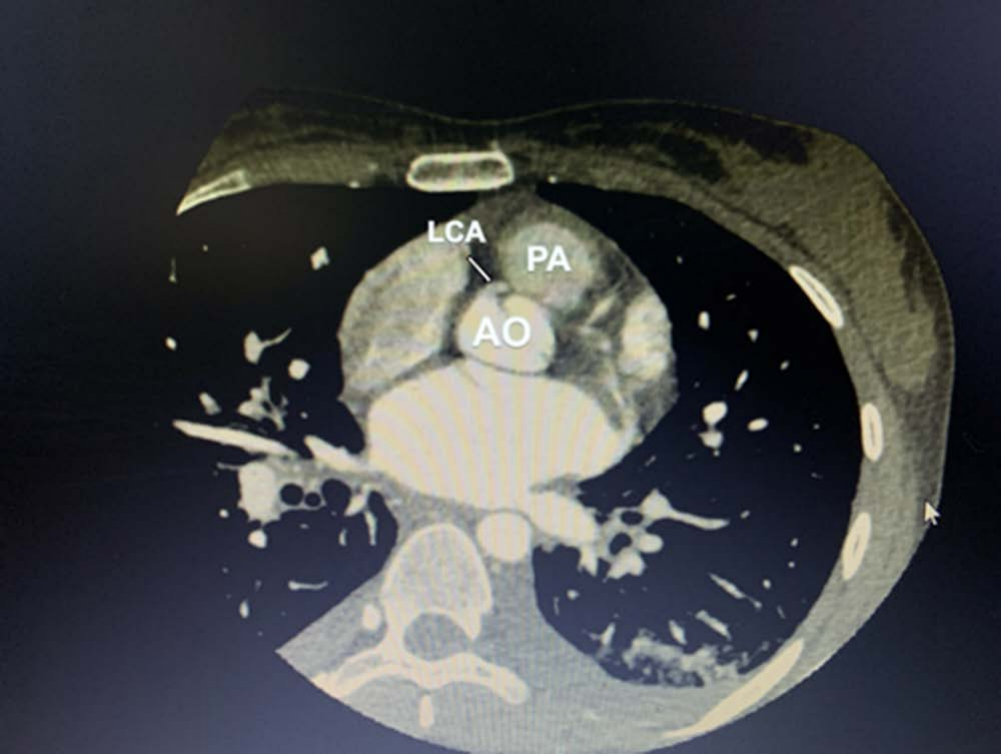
Coronary CTA: LCA is located between the Ao and the Pa according to axial section, with originating from the right sinus and exhibiting intramural course.
